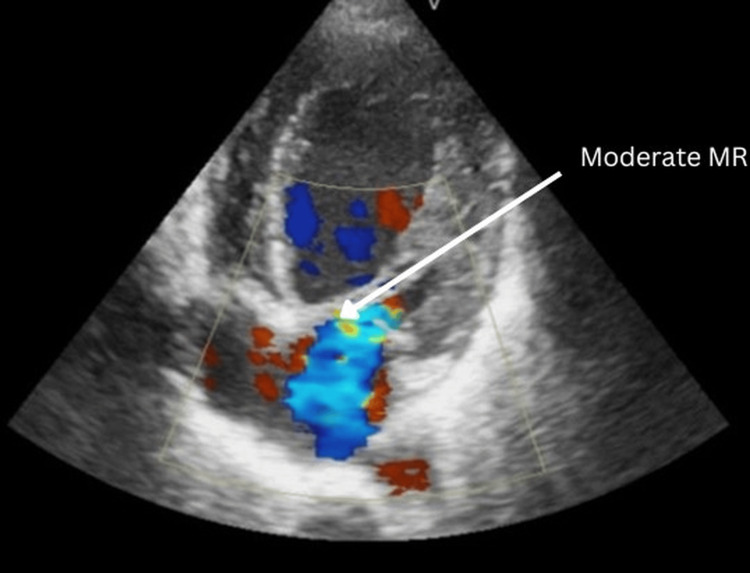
Transthoracic echocardiography (apical four-chamber view) - image 2 Color Doppler image demonstrating a dilated left ventricle with grade II mitral regurgitation. Chamber labels (RV, RA, LV, and LA) are provided; arrows highlight the dilated LV/LA and the moderate mitral regurgitation jet RV: right ventricle; RA: right atrium; LV: left ventricle; LA: left atrium
